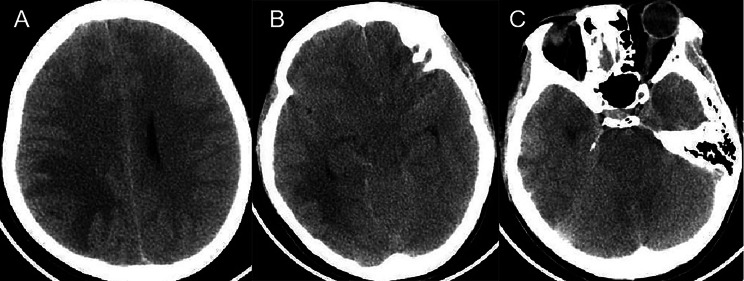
Axial cut of CT scan of the brain. A: A patchy low-density shadow was observed in the right temporal–parietal lobe. The right lateral ventricle was compressed and flattened. B:The suprasellar cistern was compressed and narrowed, displaying an irregular shape. Brain herniation needed to be excluded. C: The brainstem was compressed, and the boundaries were unclear. Brain swelling may have occurred
